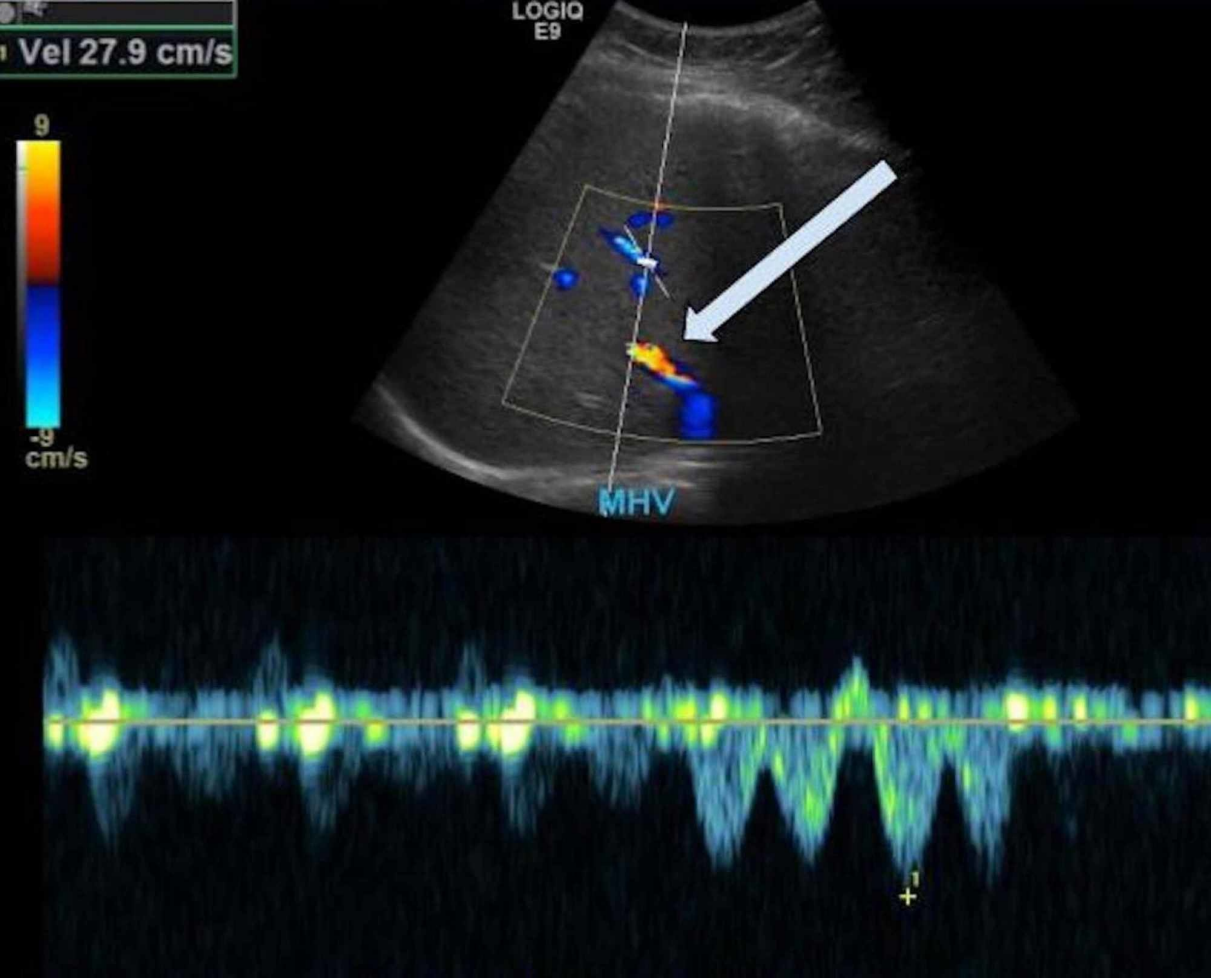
Ultrasound abdominal Doppler (liver portal-venous system) Hepatomegaly and normal Doppler ultrasound examination of the hepatic vessels. Middle hepatic vein (MHV) velocity (Vel) is 27.9 cm/s (arrow). Patent with normal waveform.
